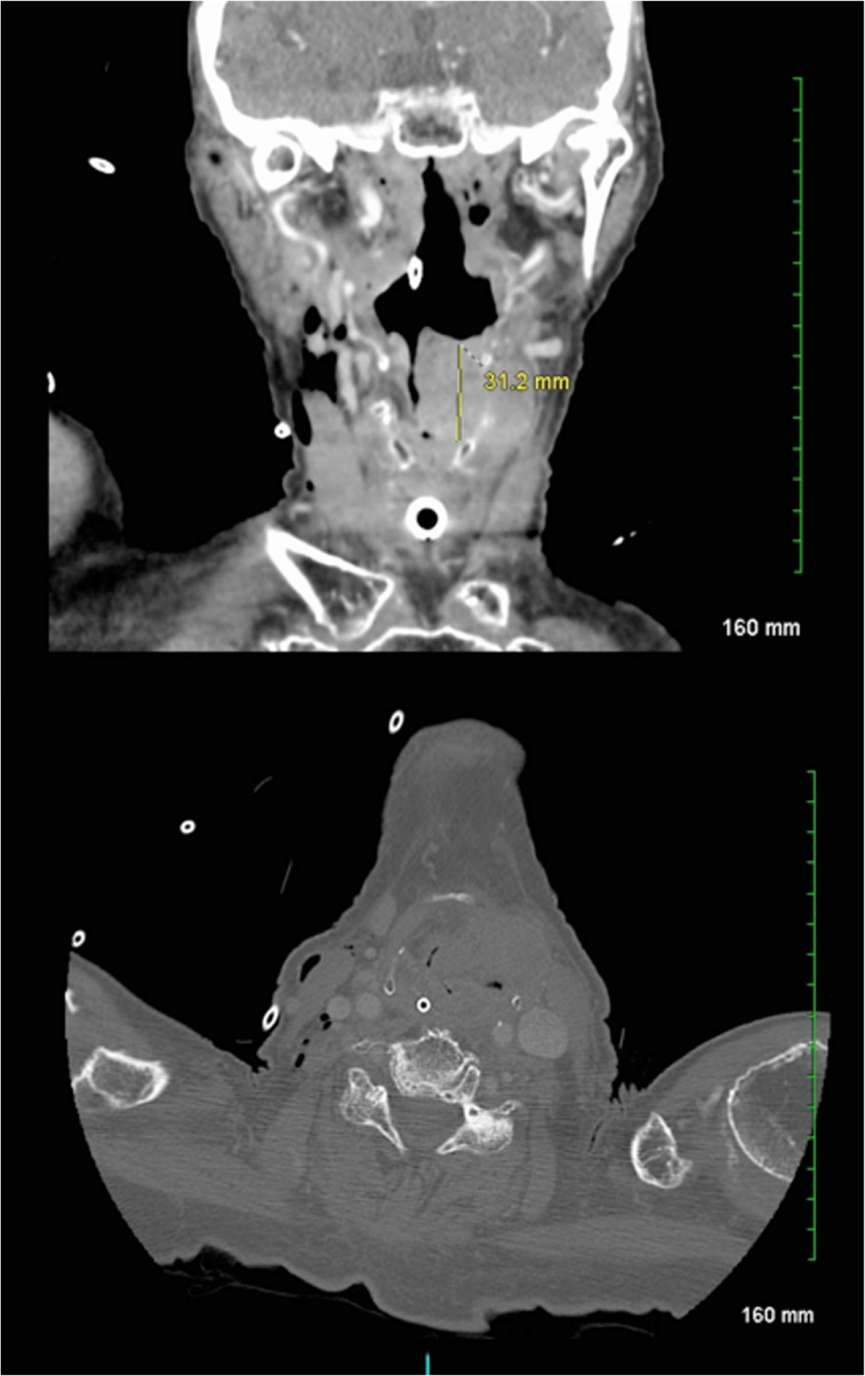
Initial computed tomography scan of the patient’s neck showing left neck mass involving the left supraglottic larynx and extending to the glottis and paraglottic space. The mass extended out through the thyrohyoid membrane and involved the left strap muscle
